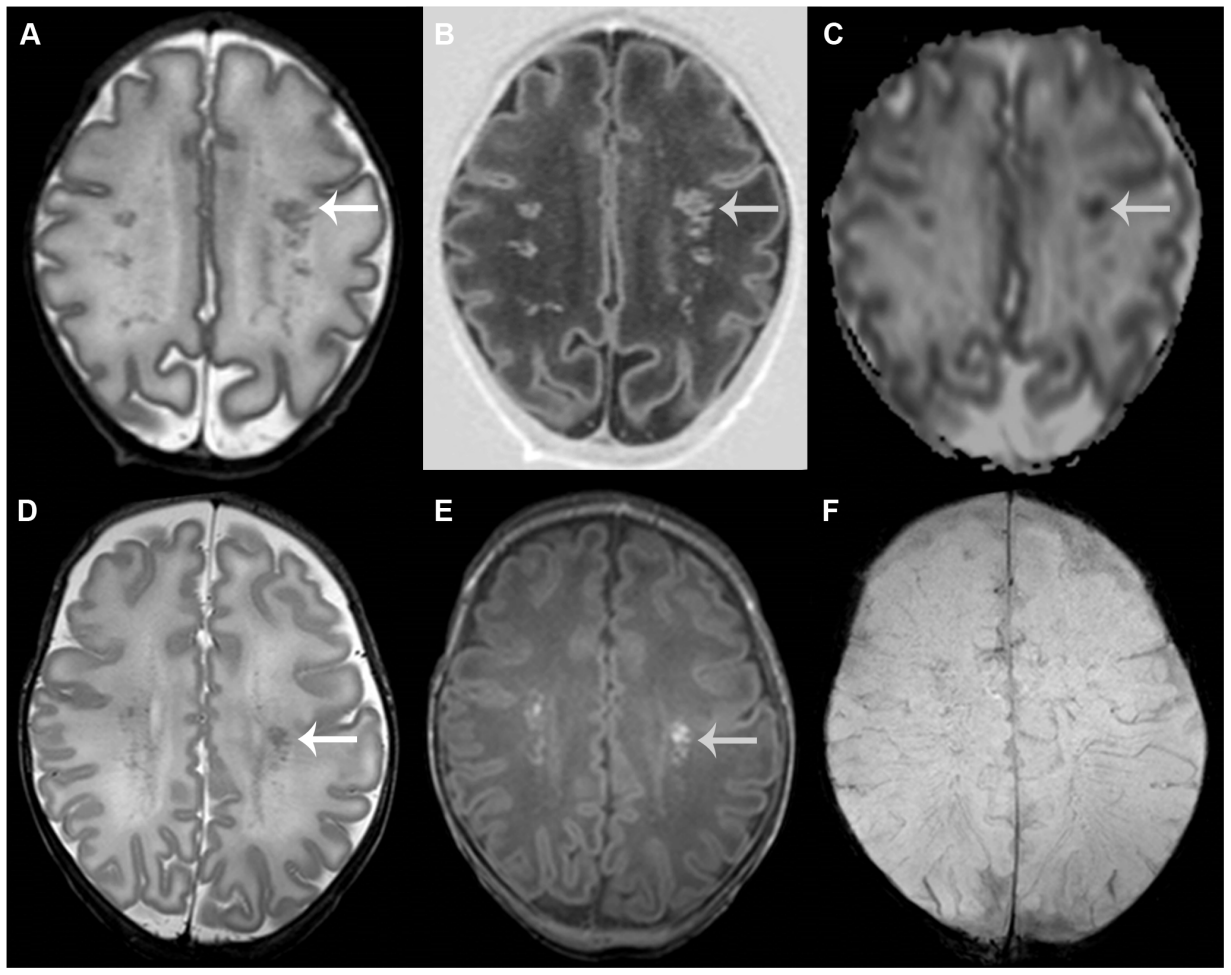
Punctate white matter lesions with a cluster pattern. Early (A–C) and term equivalent (D–F) scans in an infant of 31+3 weeks gestation with lesions in a cluster pattern. T2-weighted imaging shows multiple lesions throughout the white matter (A) that are also clearly visible on inversion recovery imaging (B). The apparent diffusion coefficient -map shows restricted diffusion at the site of the lesions (C). The SWI sequence (not shown) did not show any signal loss. The arrow indicates one of the lesions, visible on all images. At term equivalent age, lesion load has diminished. Lesions are now better appreciated on T1-weighted imaging (E), compared with T2-weighted imaging (D). Again, SWI does not show signal loss (F). Outcome was favorable with a developmental quotient of 94 on the Griffiths scales at 18 months corrected age.
